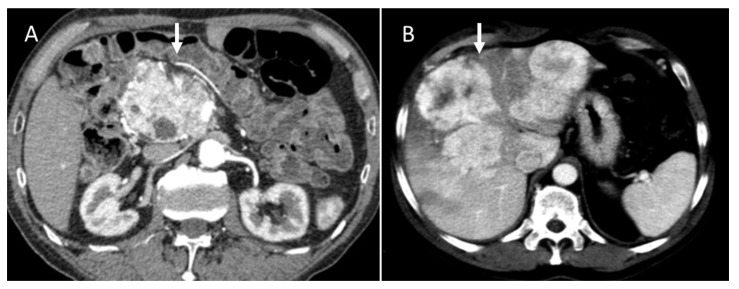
CT evaluation during arterial phase of pancreatic NEN ((A): arrow) with liver metastases ((B): arrow).
